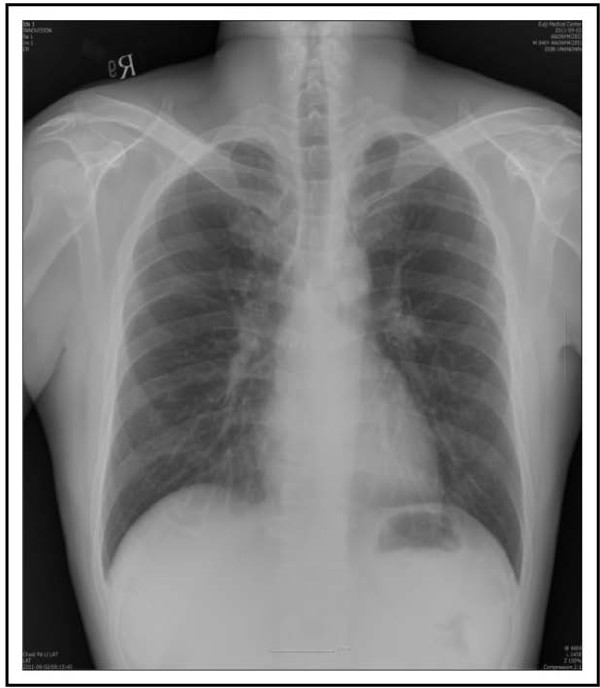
After anti-tuberculosis medication, chest X-ray showed improvement.
